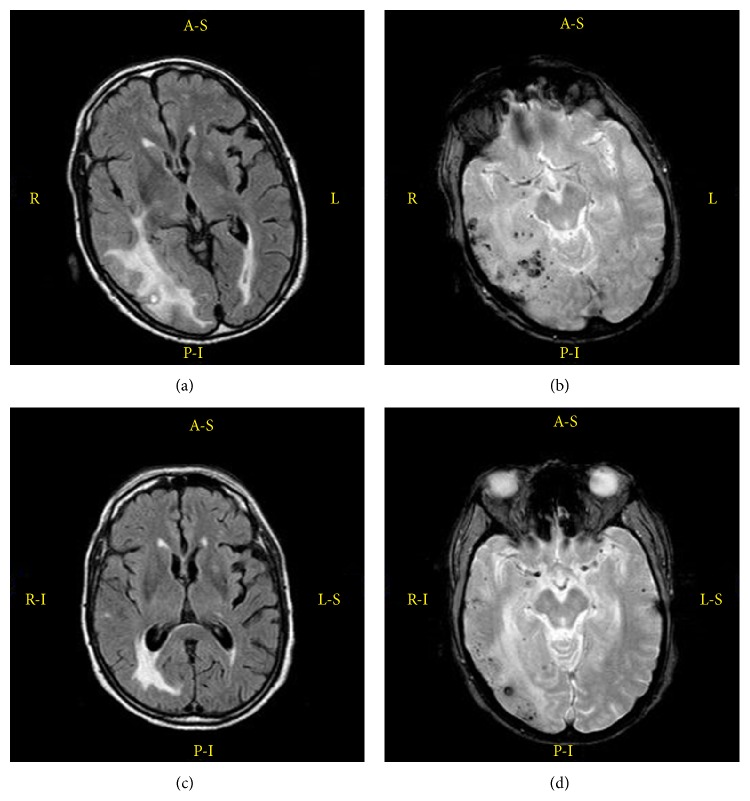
MRI findings of CAA-ri. Axial FLAIR images revealing bilateral hyperintense lesions (a). GRE images showing cortical-subcortical microhemorrhages (b). After one month of steroid therapy MRI sequences revealing the reduction of both cerebral edema and microhemorrhages (c-d).
